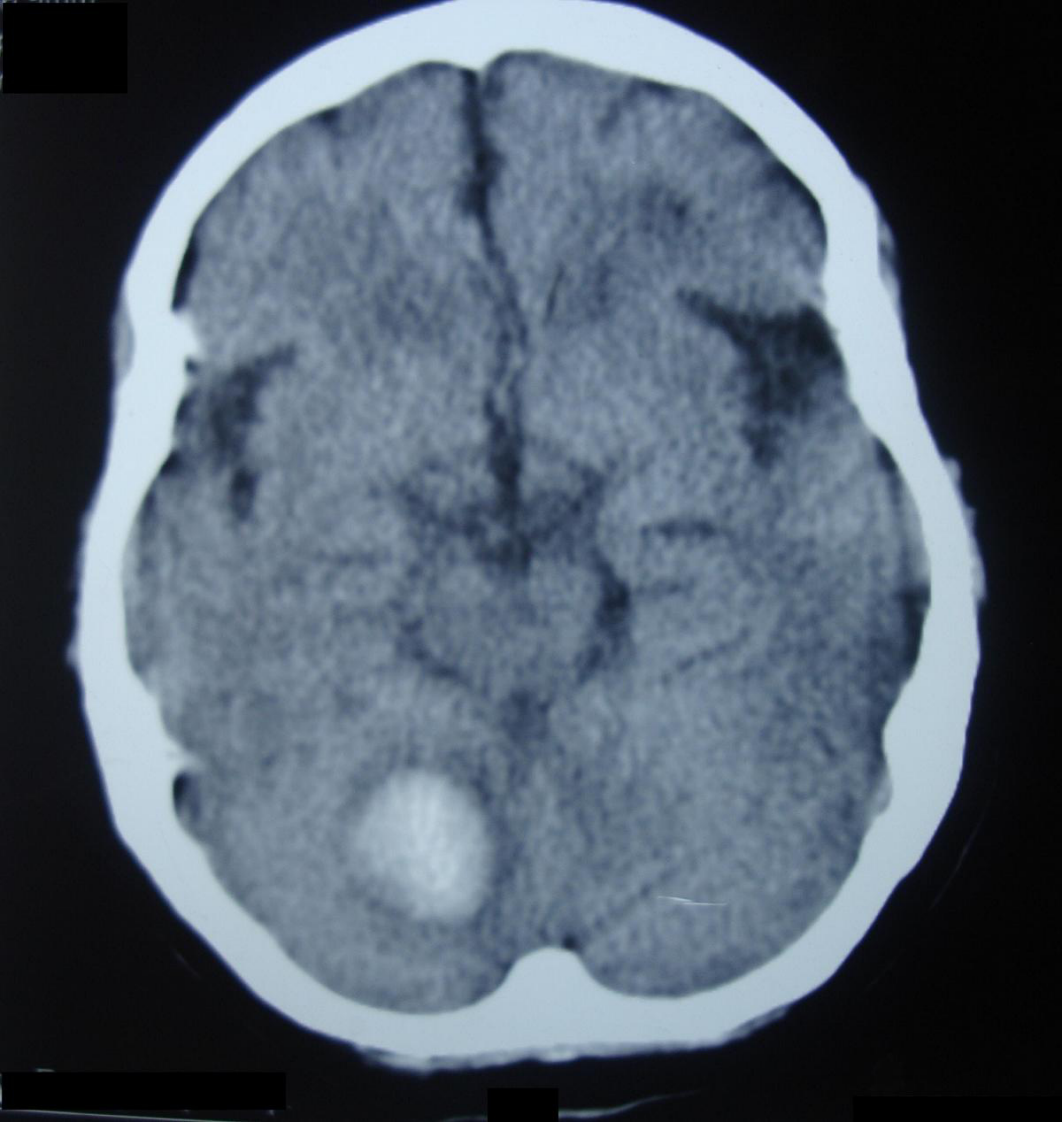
Non-contrast brain CT scan of the patient, which was done approximately four hours after developing her symptoms. Note that the hematoma lies at the deep right cerebellar hemisphere and is surrounded by mild cytotoxic edema. The fourth ventricle was not compressed and brainstem signs were absent.
